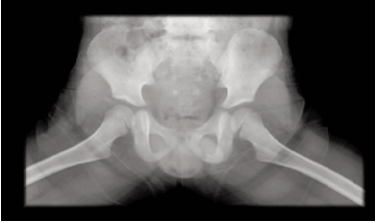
Fig. 2: MRI of both hips with contrast T1 coronal image with contrast -, showing abscesses (rim enhancing lesions (A) arising from the inner aspect of left ischium and crossing the ischium (I). N= neck of femur. F2:
